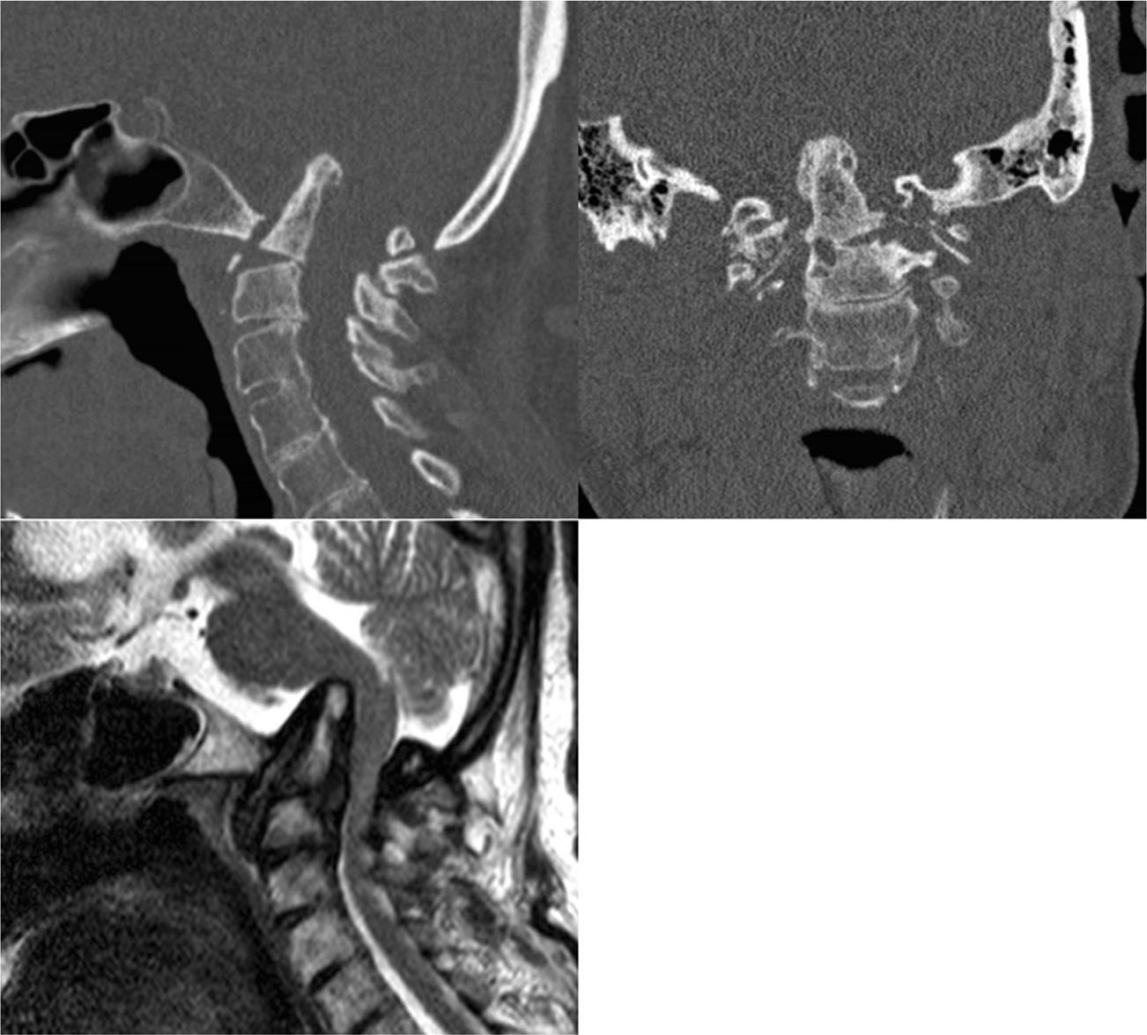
Basilar invagination in a 62-year-old female. Computed tomography, sagittal (a) and coronal (b) planes; magnetic resonance imaging, sagittal plane (c)
